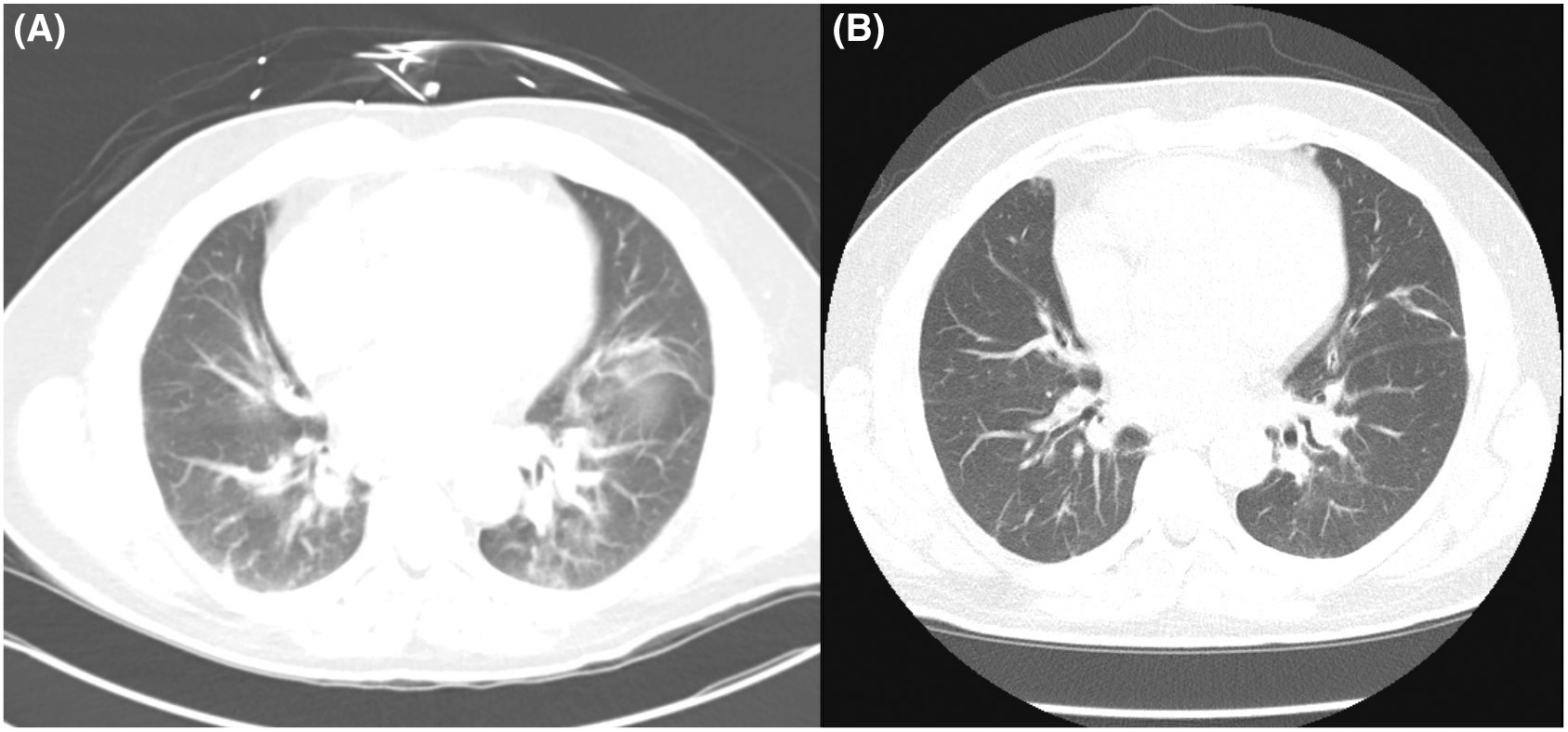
CT scan of the chest (A) before treatment and (B) after 5 months of treatment with improvement on prednisone taper and mycophenolate mofetil.
